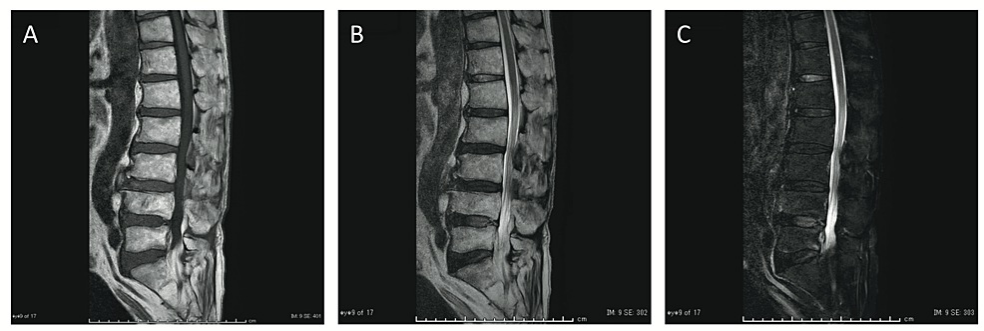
Lumbar spinal cord MRI of Case 4 Τ1-weighted image (A), T2-weighted image (B) and fat-suppressed T2-weighted image (C) of the lumbar spinal cord MRI showed no abnormal findings, including significant canal stenosis, abnormal signal intensity in the spinal cord, or cauda equina.
